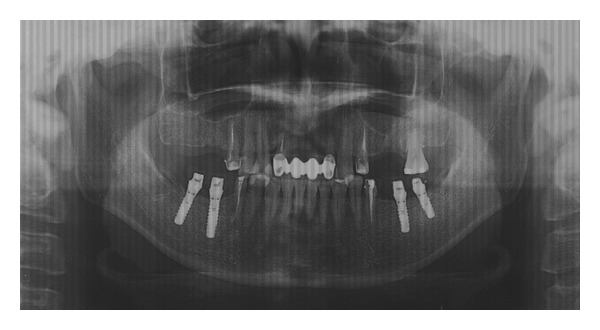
Panoramic radiograph shows a case of atypical odontalgia causing in a 57-year-old woman. The woman complained of toothache in the bilateral upper molars after the replacement of dental implant into the lower jaws. The root canal treatments of upper molars were performed, and the upper molars were finally extracted, since her pain was not relived by any treatments. A prescription of 40 mg per a day of amitriptyline decreased symptoms in the patient.
